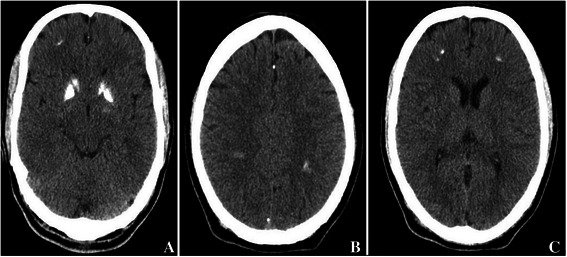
CT scan brain showing extensive intracranial calcifications involving basal ganglia (a), occipito-temporal (b) and fronto-parietal lobes (c)
